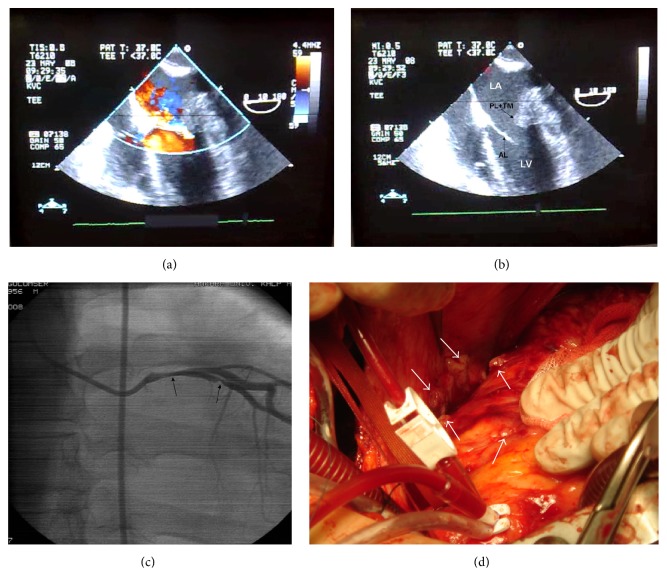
((a) and (b)) Transesophageal echocardiogram midesophageal 4-chamber view at 0°. (a) Color Doppler image shows severe (III/IV) mitral regurgitation. (b) The two-dimensional image shows an echogenic mass in the left atrium, arising from the region of the posterior mitral leaflet and causing restriction of the movements of this leaflet. (c) Coronary angiogram (left anterior oblique view with caudal angulation) showing significant stenoses at the left main (70%) and circumflex (80%) arteries (arrows). (d) Intraoperative view of the pericardial space. Extensive and multiple masses in the pericardium and multifocal central hemorrhage in the epicardium are shown.
